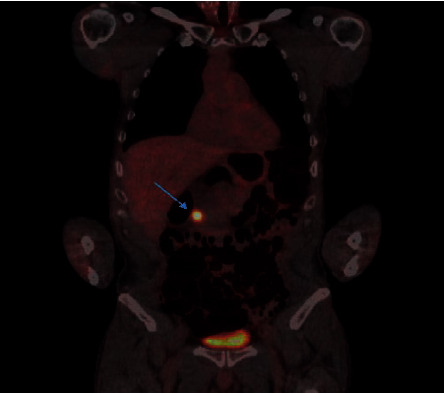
Positron emission tomography-computed tomography scan showing a focus of intense activity corresponding to the area of the pancreatic head (blue arrow) without a clear anatomical correlate.
